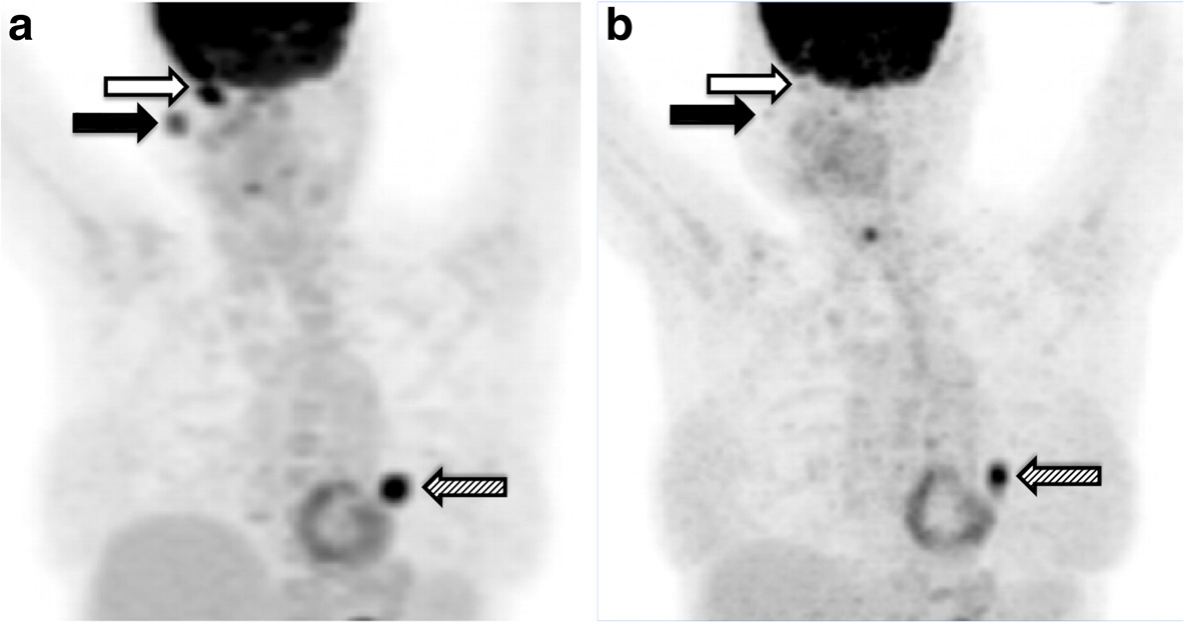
a FDG-PET/CT in December 2012, prior to BRAF inhibitor therapy, showed intensely FDG avid lesions in the orbit, the parotid glad and the lung post AdCD40L treatment. b PET/CT in May 2013, after the initiation of the treatment with vemurafenib, showed that all the previously described intensely FDG avid lesions had lower SUVmax uptake. Physiologic FDG uptake in right posterior vocal cord is observed. Black arrow: metastasis in right parotid gland. White arrow: metastasis in right orbit. Striped arrow: lung metastasis
